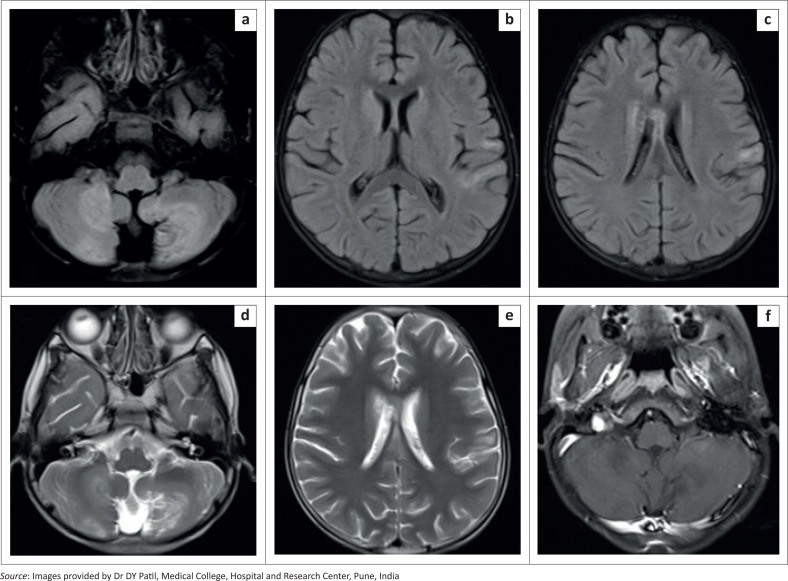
[Case 2]: Brain MRI in a 10-year-old male showing multiple patchy FLAIR (a-c) and T2 hyperintense areas of altered signal intensity in the cerebellar hemispheres, left temporoparietal lobe and body of the corpus callosum, (d, e) with no post-contrast enhancement (f). Subtle FLAIR hyperintense signals were also seen in the inferior part of the medulla (a).
